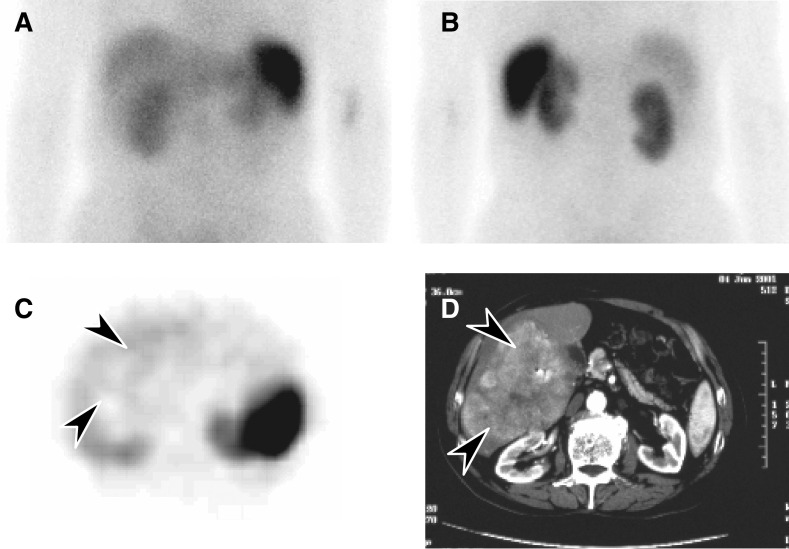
(A) Octreotide scintigraphy (4-h image) showing no evidence of uptake in an HCC in the right lobe of the liver: anterior image; (B) posterior image; (C) SPECT transaxial section through the upper abdomen showing reduced uptake in the HCC (arrows) compared with normal liver; and (D) the HCC in a corresponding CT scan slice (arrows).
